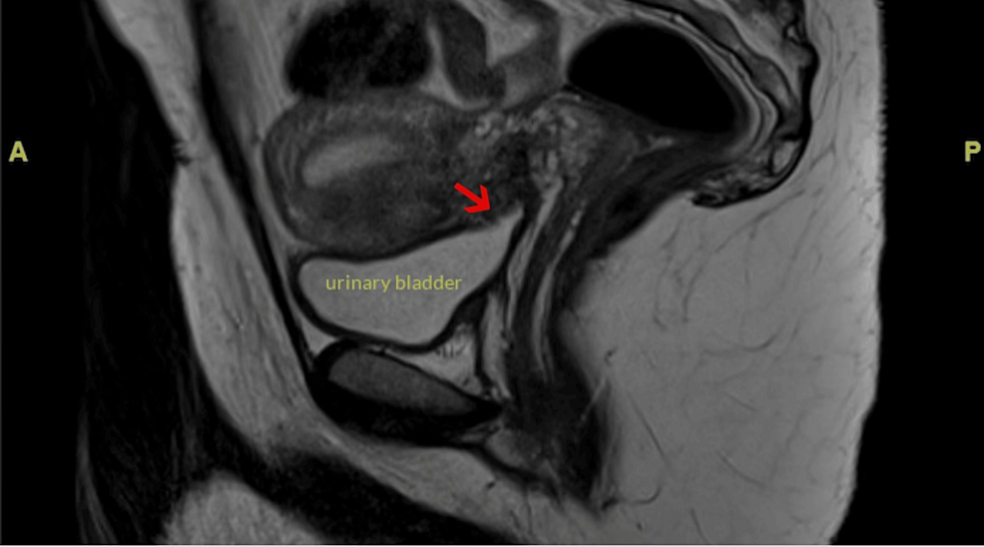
Midline sagittal T2 weighted magnetic resonance image through the pelvis demonstrating chorionic tissue extending beyond the serosal margin of the myometrium and being inseparable from the serosal margin of the urinary bladder dome (red arrow).
